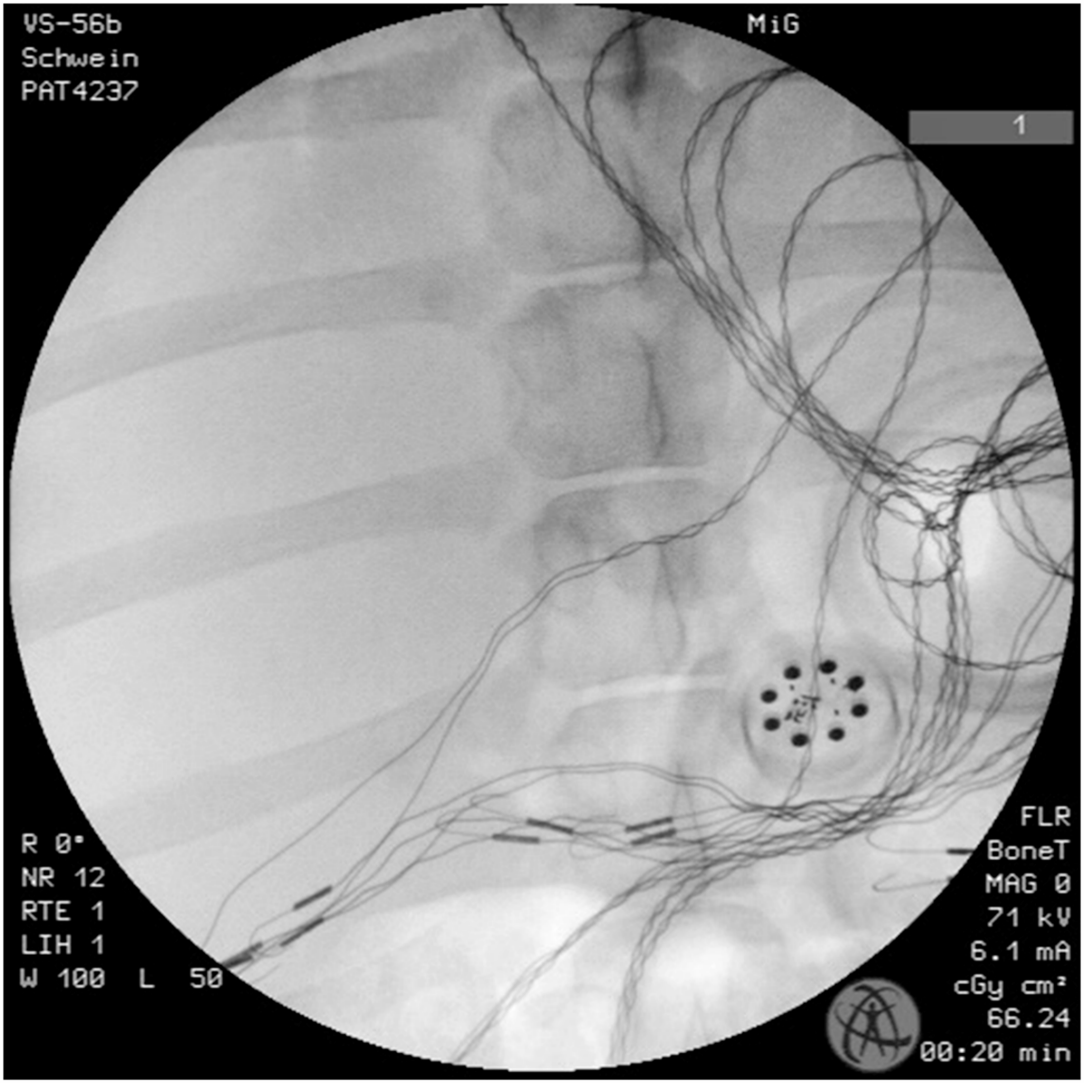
Conventional X-ray image shows the prototype positioned on the stomach. The leads of the hook electrodes for the EMG recordings are also visible
